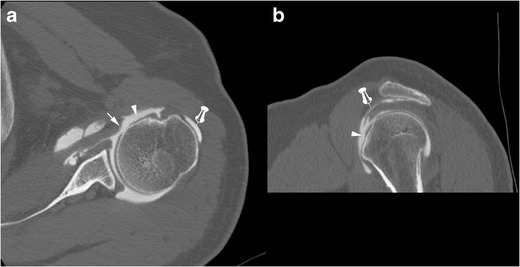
Computed tomography athrography of the left shoulder with axial (a) and sagittal oblique (b) multiplanar reconstructions. Opacification of the SASD (pin) and whole disruption, either laterally (a) or vertically (b) (arrow heads) of the SSC tendon are consistent with a complete full thickness tear. Intermediate retraction of the tendinous stump (arrow)
